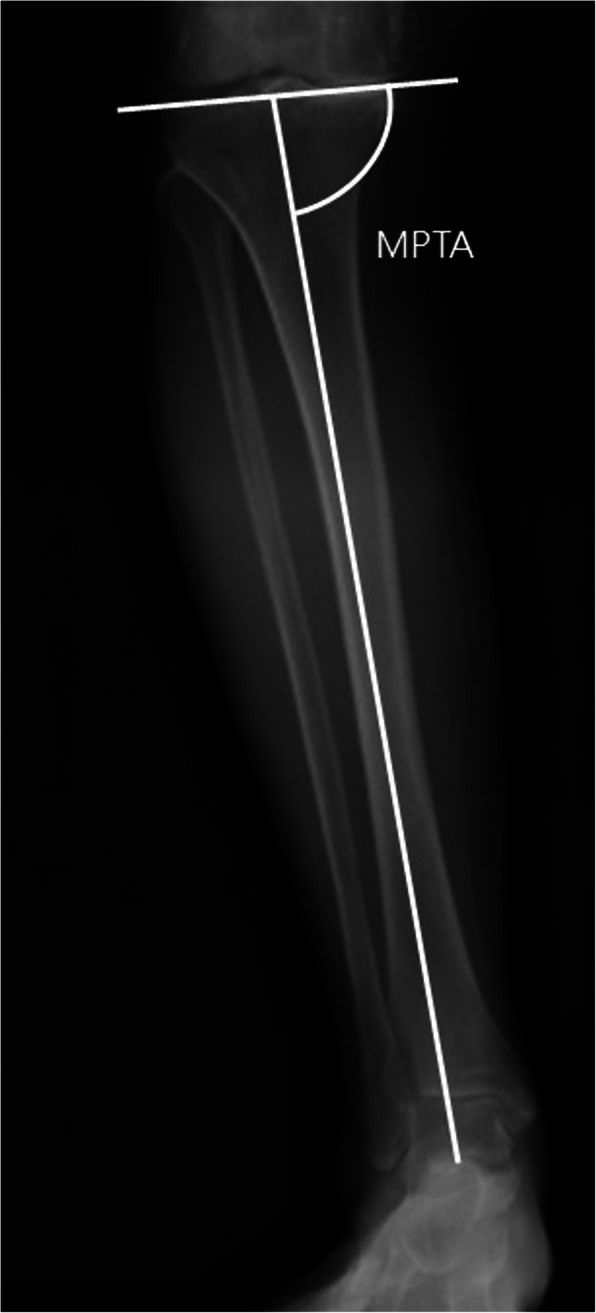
The medial tibial plateau angle (MTPA) is measured on the whole-leg weight-bearing radiograph. It is defined as the medial angle between the tibial articular marginal line and the mechanical axis line of the tibia (line from the ankle center to the center of the tibial spines)
